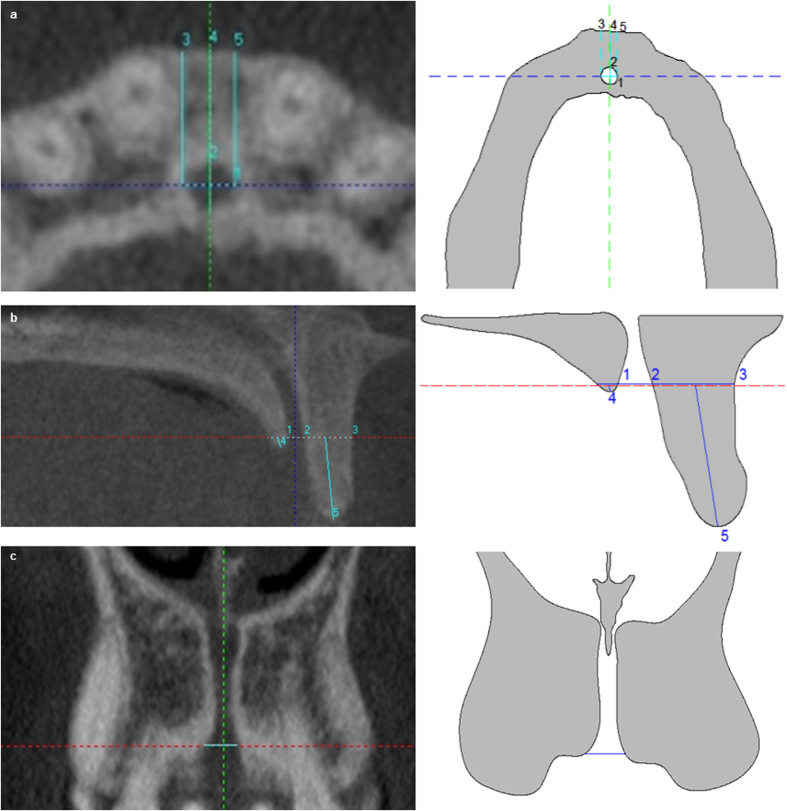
Radiographies and draw-scheme of level 1 show the follows: (a) Axial slice: (1) bpD1, (2) tD1, (3) LW1, (4) CW1 and (5) RW1. (b). Sagittal slice: (1) PW1, (2) SD1, (3) BW1, (4) PL1 and (5) BL1. (c) Coronal slice: CD1.
